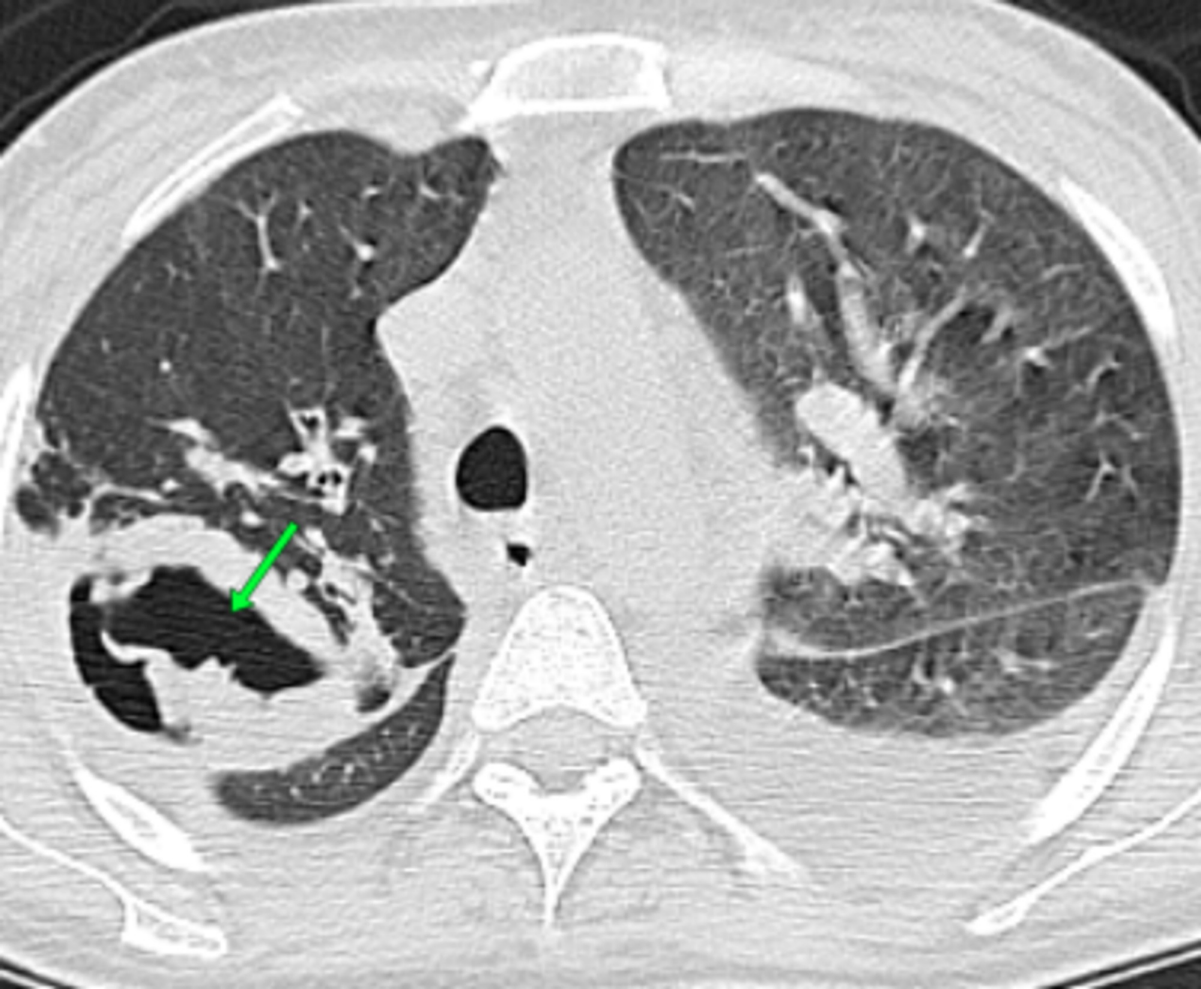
Chest CT scan showing a large excavated lesion caused by aspergillus in the right lung (arrow) CT: computed tomography
